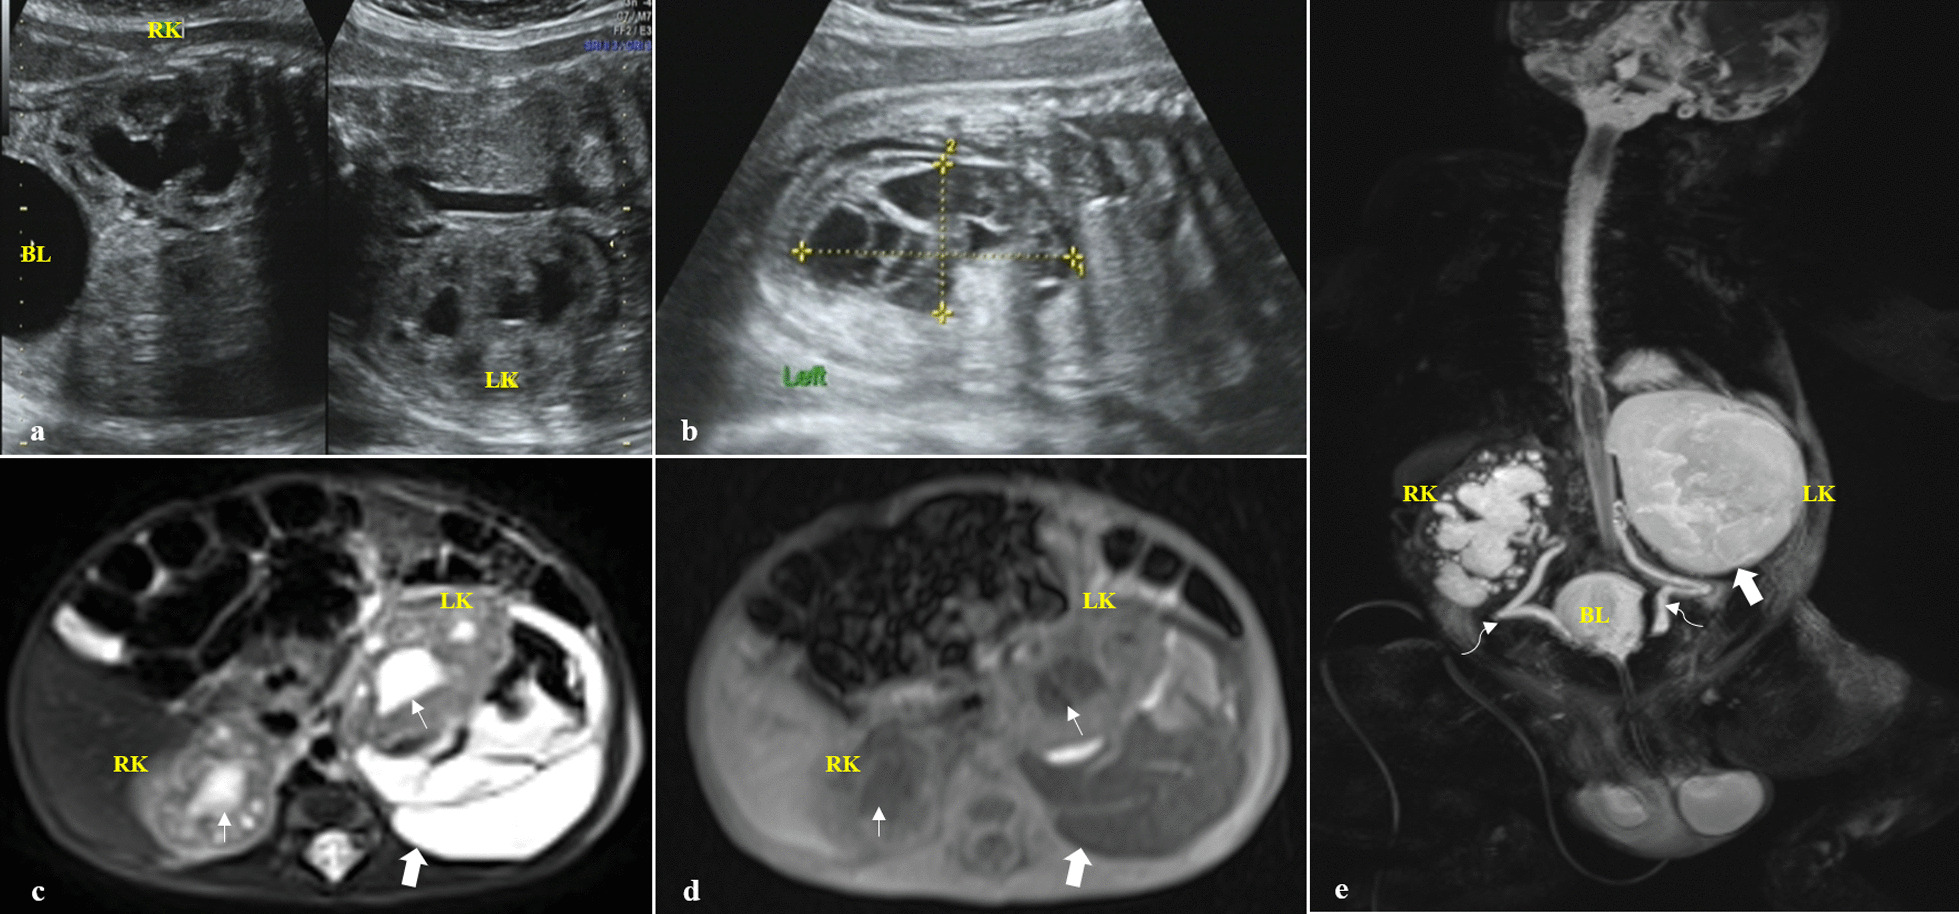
a and b Coronal view of ultrasound showing bilateral hydronephrosis and the left WS at 36 weeks of gestational age. c and d Cross-weighted images of T2 and T1 by postnatal infant MRI demonstrating bilateral hydronephrosis and WS, respectively. e MRU imaging of the urinary system revealed a thickened urinary bladder and bilateral ureteral dilatation. Thick and thin arrows show WS and hydronephrosis, respectively. Curved arrow showing ureter dilatation
